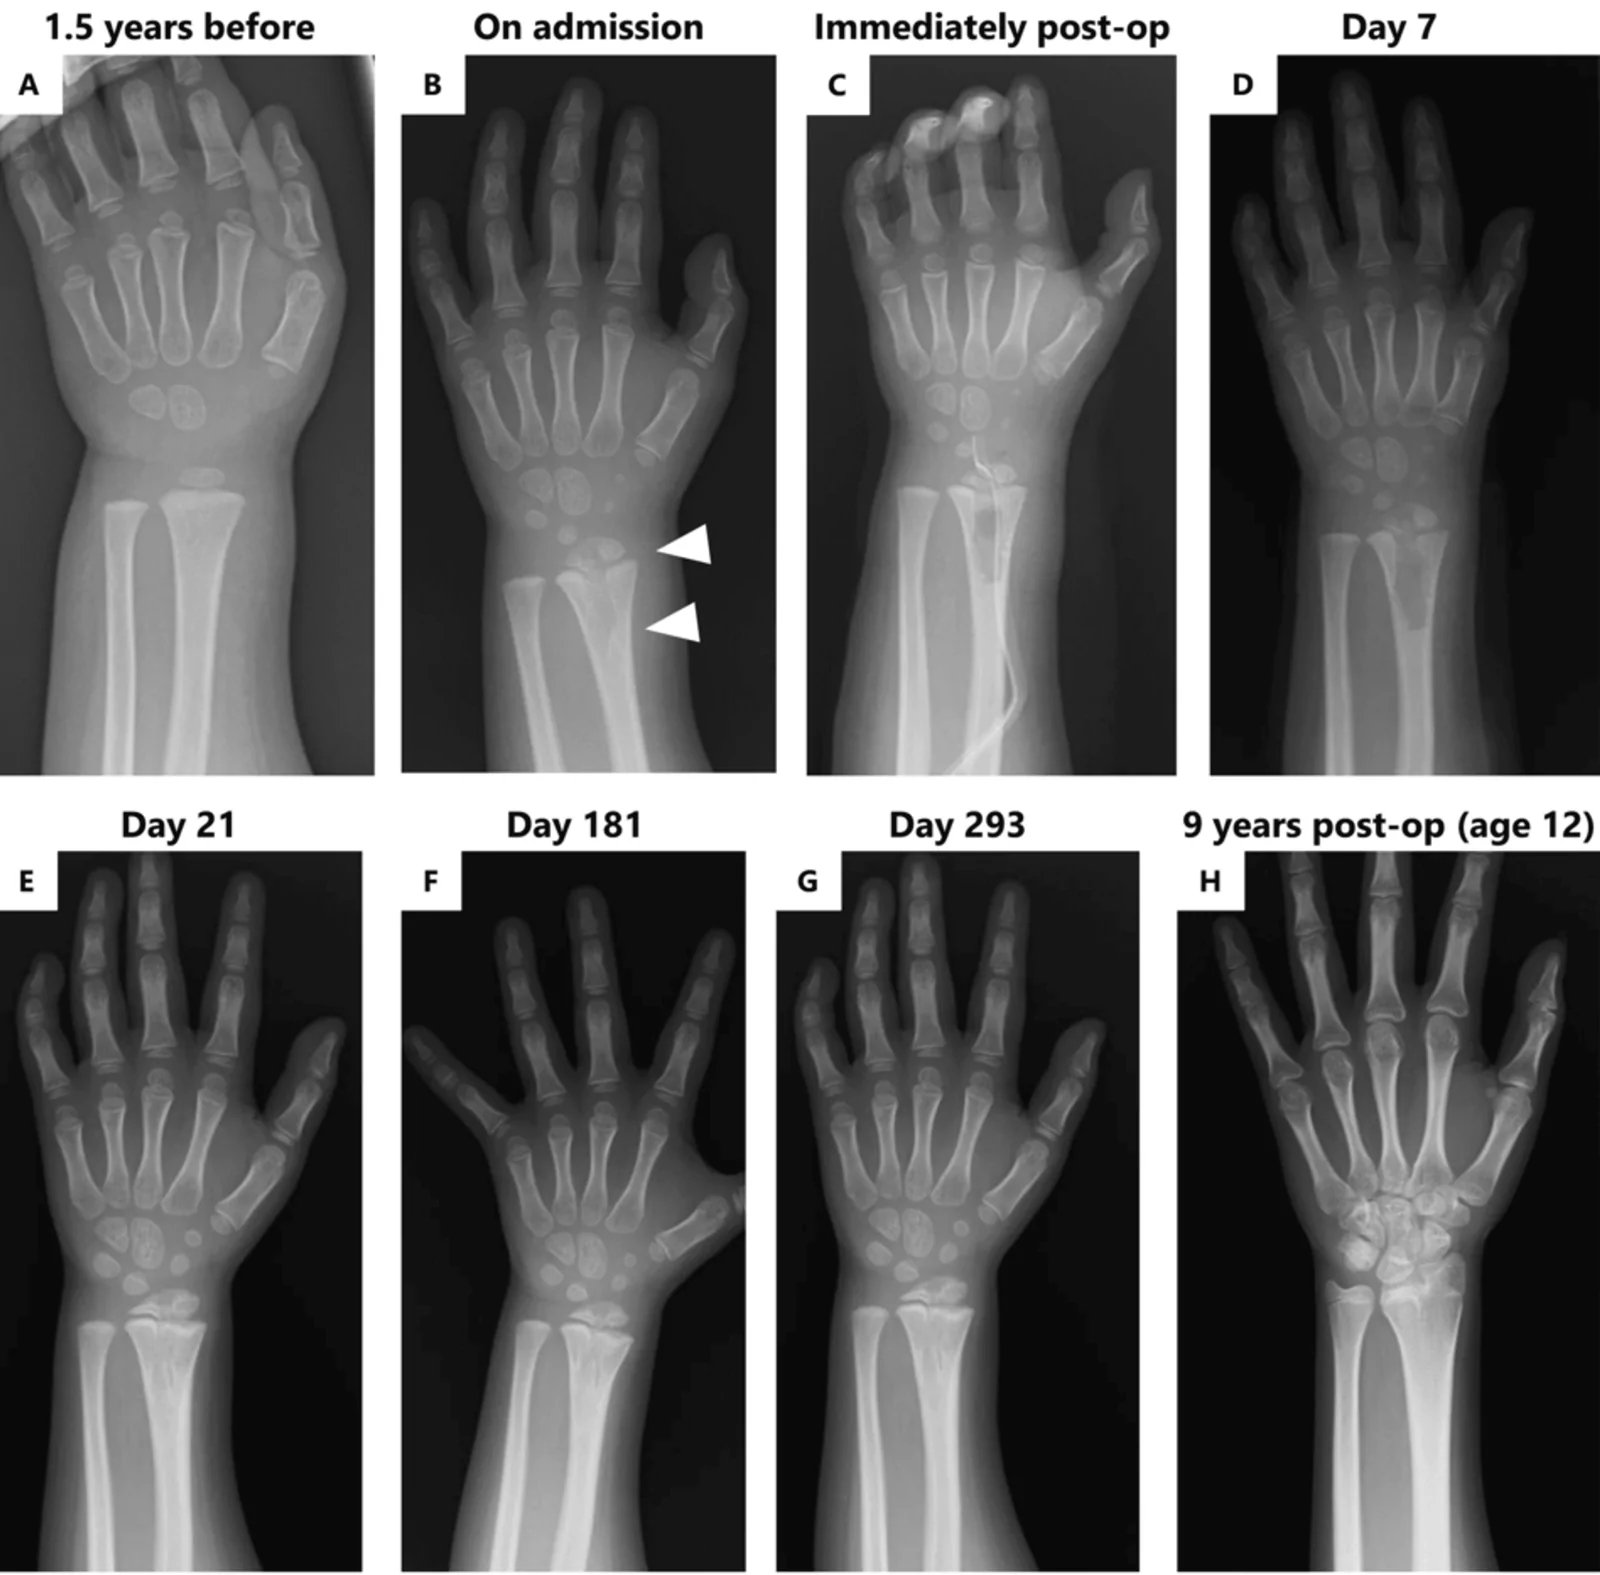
Serial plain radiographs of the left forearm Images are displayed with the distal end (i.e., fingertips) at the top. (A) 1.5 years before admission (at the time of the cellulitis episode): no bone abnormality. (B) On admission: soft-tissue swelling over the volar surface of the distal radius and osteolytic lesions spanning the distal radial metaphysis and epiphyseal nucleus across the epiphyseal line (arrowheads). (C) Immediately postoperatively. (D) Hospital day 7. (E) Hospital day 21. (F) Hospital day 181 after surgery. (G) Hospital day 293. (H) Nine years after surgery (age 12 years): partial closure of the radial epiphyseal line on the radial side without angular deformity
